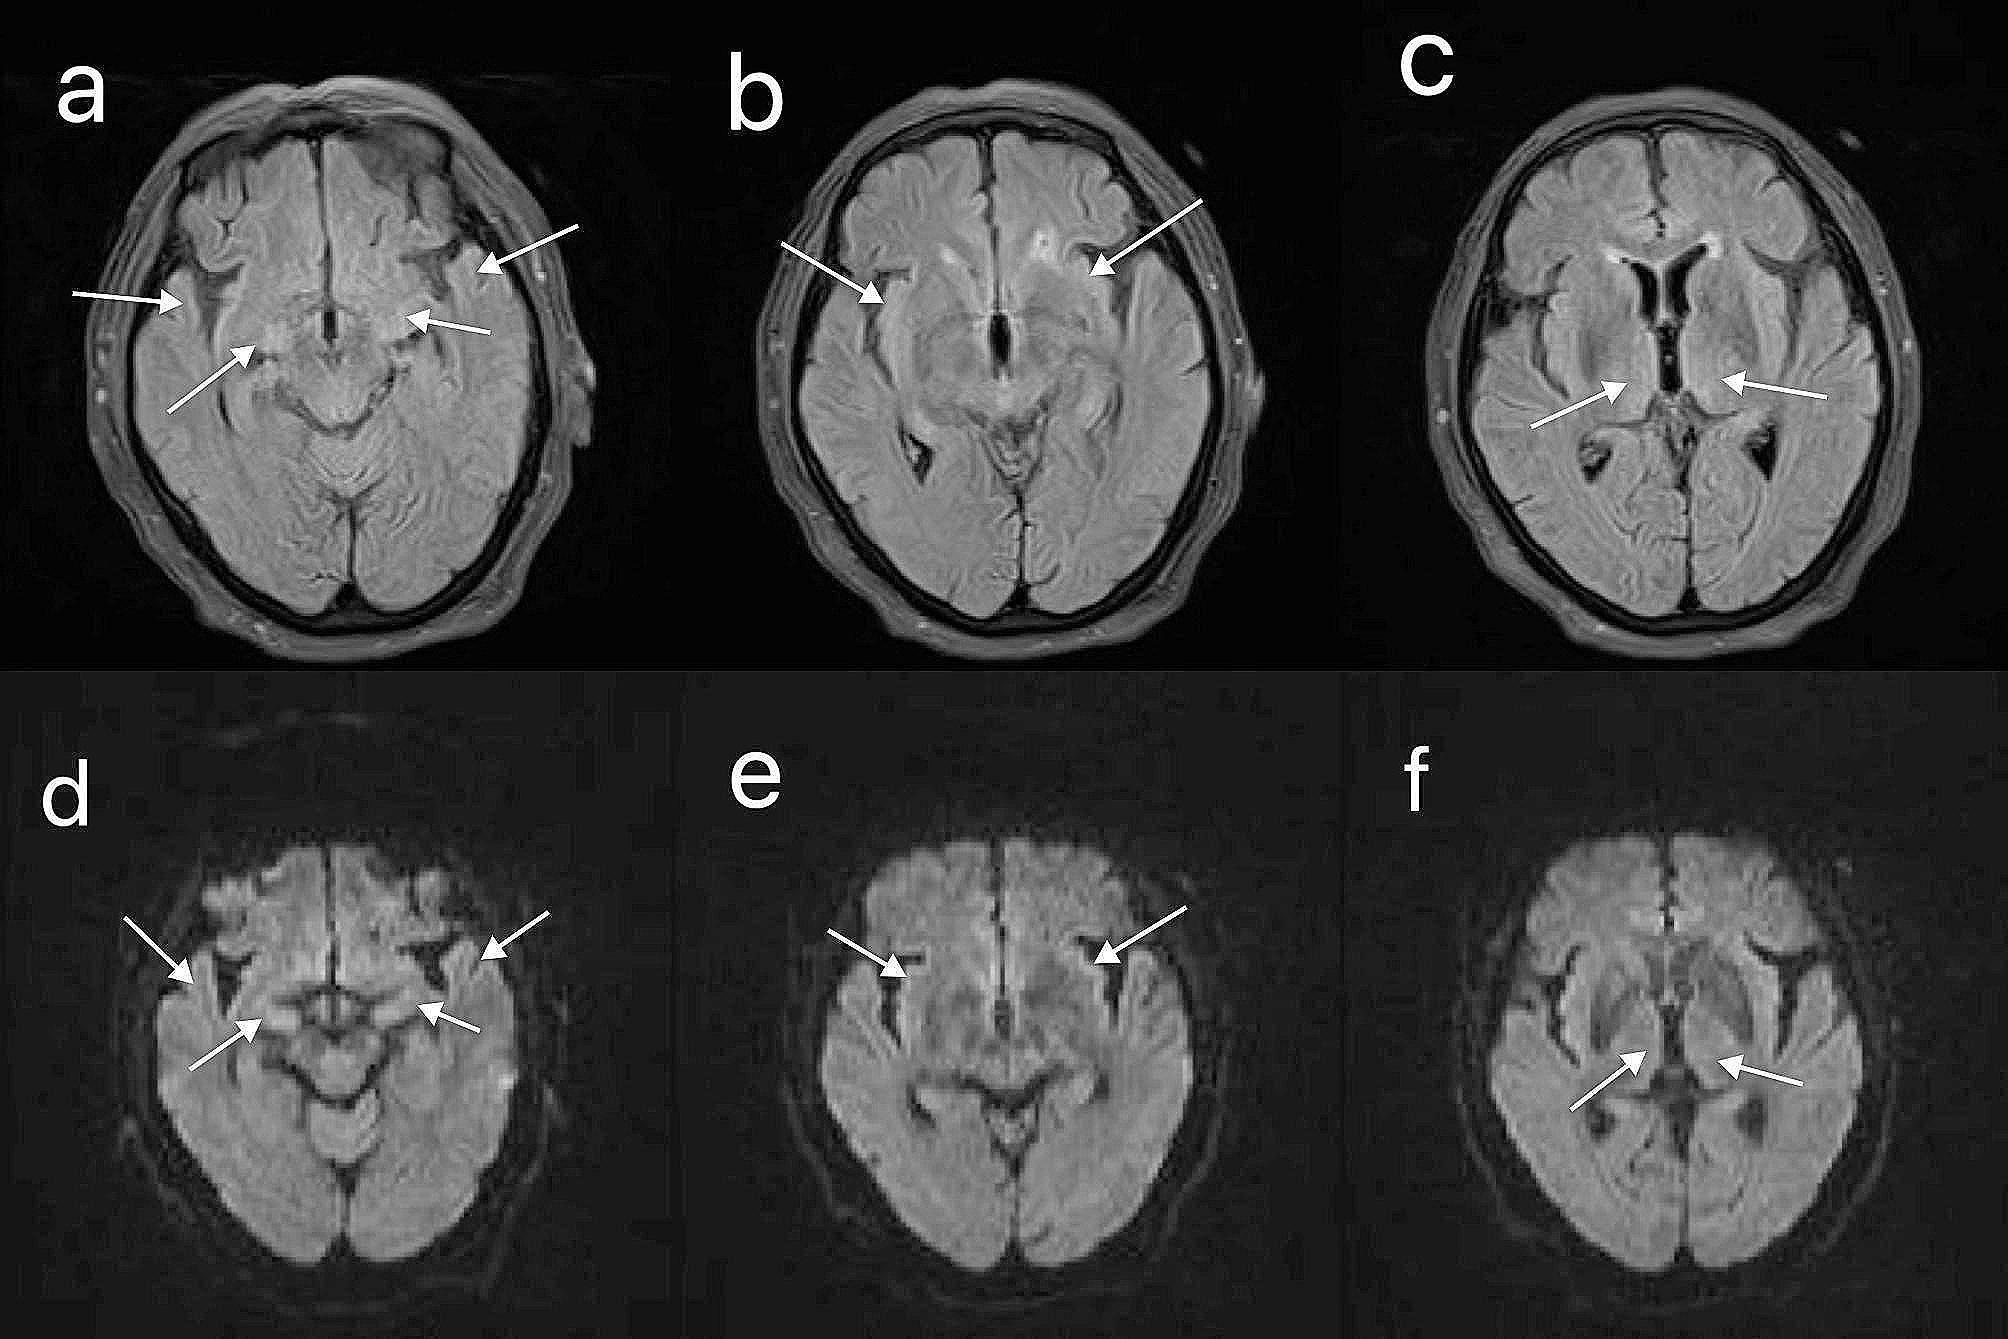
Cranial MRI of the patient 12 days after admission. Bilateral anterior temporal lobe (a and d), temporal lobe leptomeningeal gyrus (a and d), insular cortex (b and e), and bilateral thalamus (c and f) FLAIR and DWI sequences with slightly high signals
